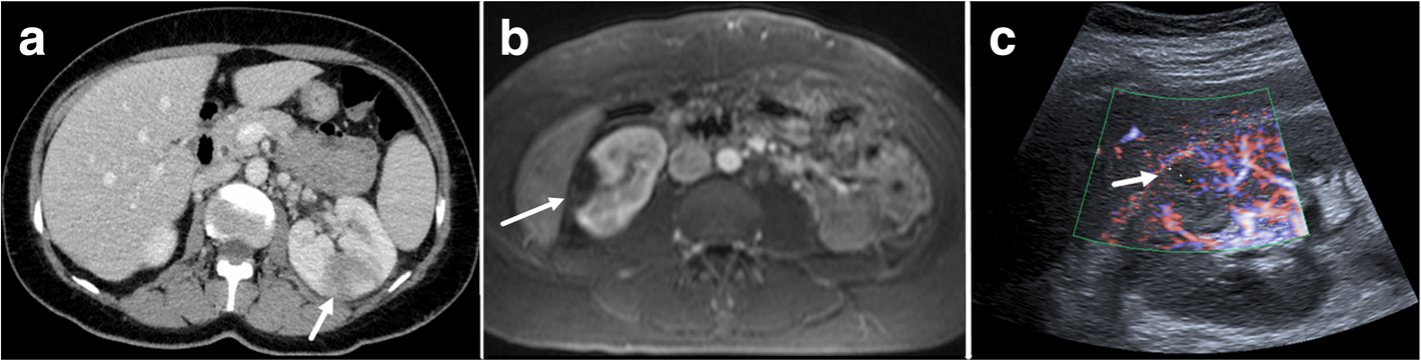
Imaging findings for AFBN. a-c Typical image findings for AFBN in CT (a), MRI (b) and Ultrasound (c). Post-contrast axial CT and MRI (T1w VIBE) showing areas of poor enhancement in the right kidney (arrows) in wedge (a) and round shape (b). Colour Doppler enhanced Ultrasound image (c) with arrow indicating focal hypoperfusion
